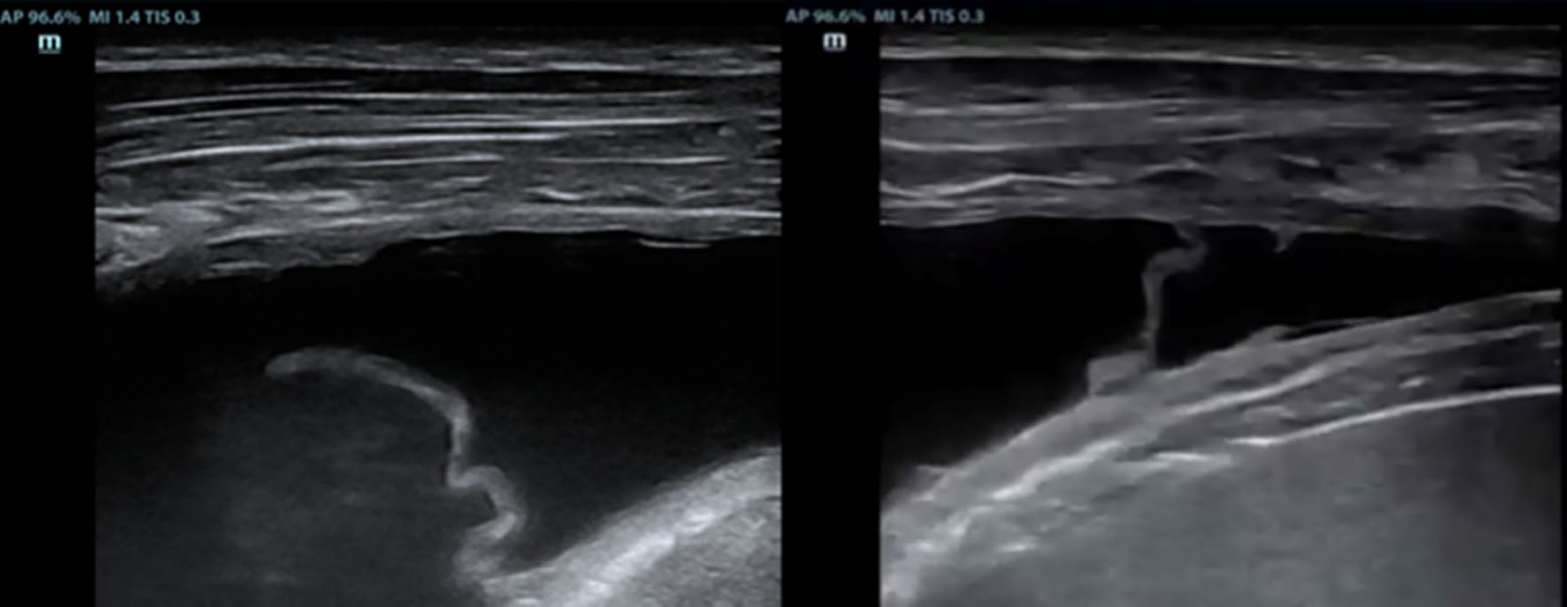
TUS showing a typical TPE. The images reveal an anechoic, free flowing pleural fluid. Thin, mobile fibrinous strand are visualized within the pleural space. Such sonographic findings, while not pathognomonic, are highly suggestive of a tuberculous etiology in the appropriate clinical context
